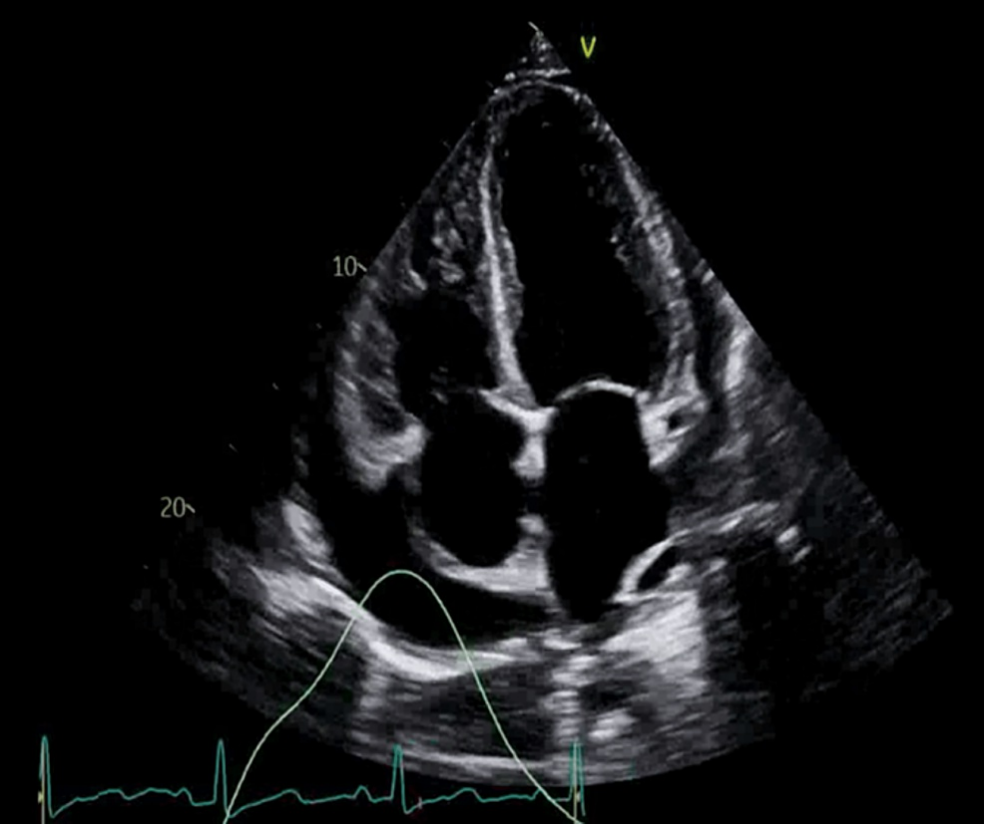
A two-dimensional echocardiogram (with different views) showing a large circumferential pericardial effusion
